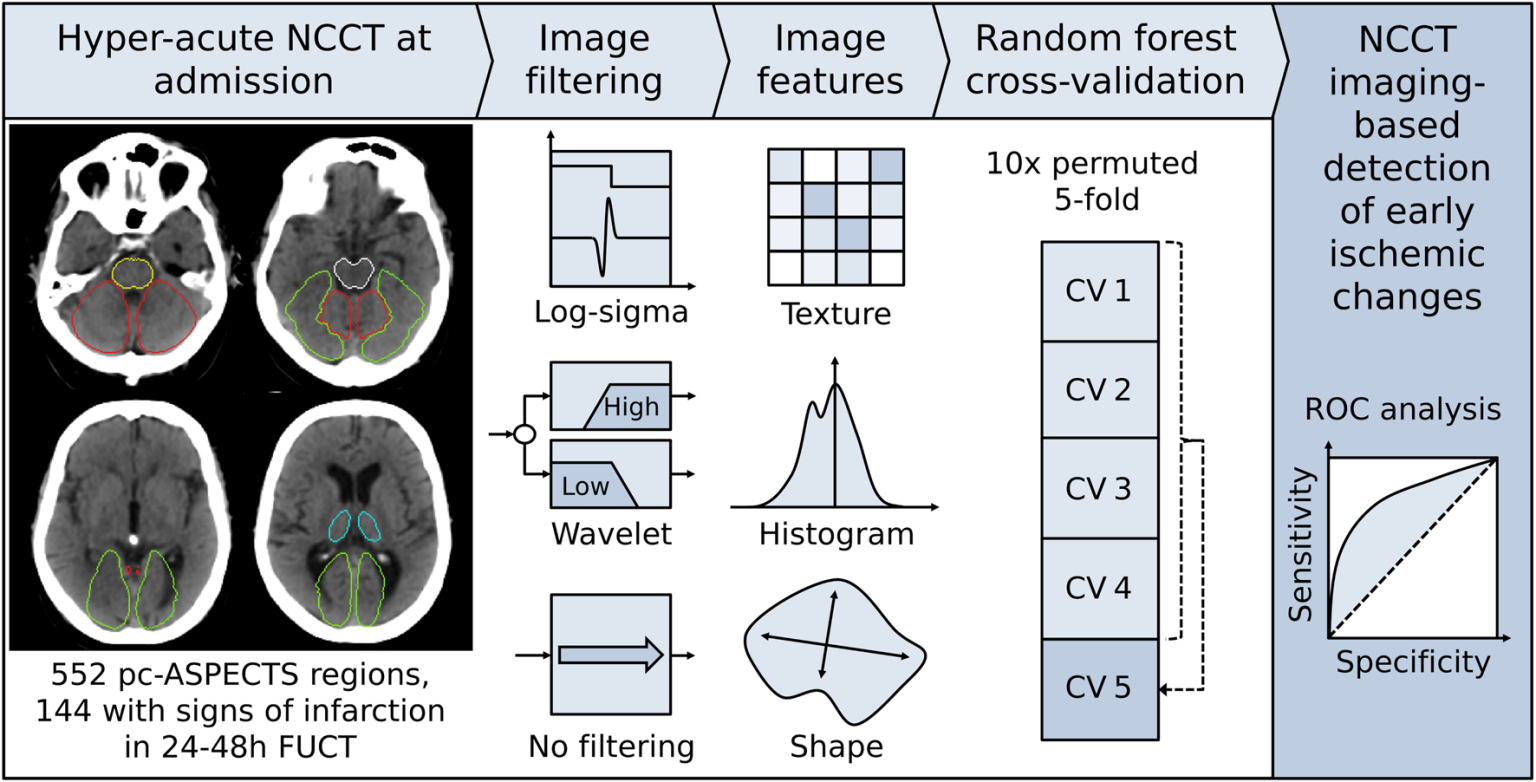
Conceptual overview of the proposed machine learning approach showing the major processing steps: CT-based image acquisition and segmentation, feature extraction (*n* = 1218), and statistical learning (random forest algorithm). *NCCT* non-contrast computed tomography, *pc-ASPECTS* Posterior circulation Acute Stroke Prognosis Early CT Score, *FUCT* follow-up computed tomography, *PCA* posterior cerebral artery
